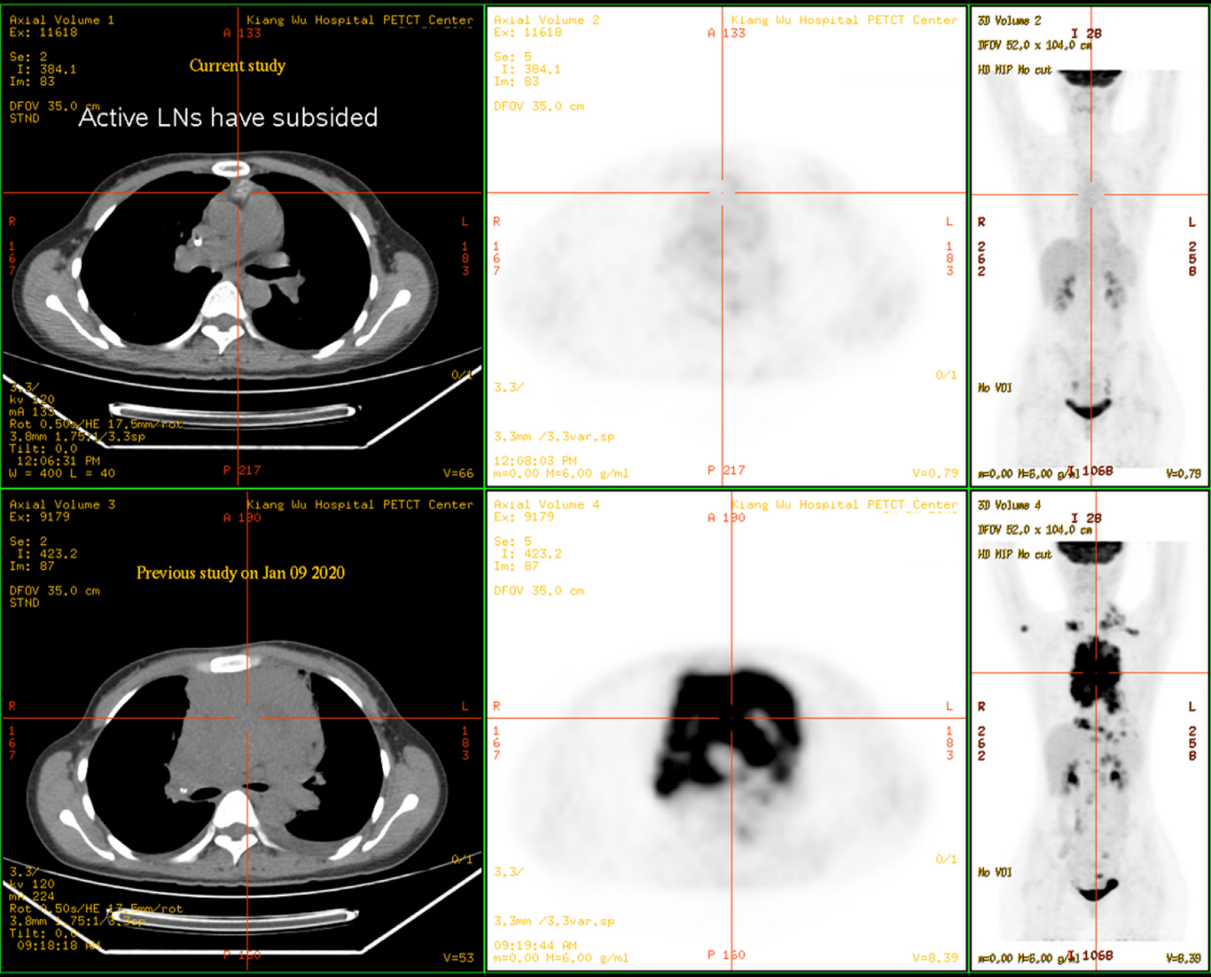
The ^18^F-FDG PET/CT scan showed complete metabolic remission. The following ^18^F-FDG PET/CT scan on September 24, 2020 indicated almost complete remission of the disease after 6 cycles R-EPOCH, 3 cycles HDMTX and 20 fractions of radiation therapy to the lung lesion.
